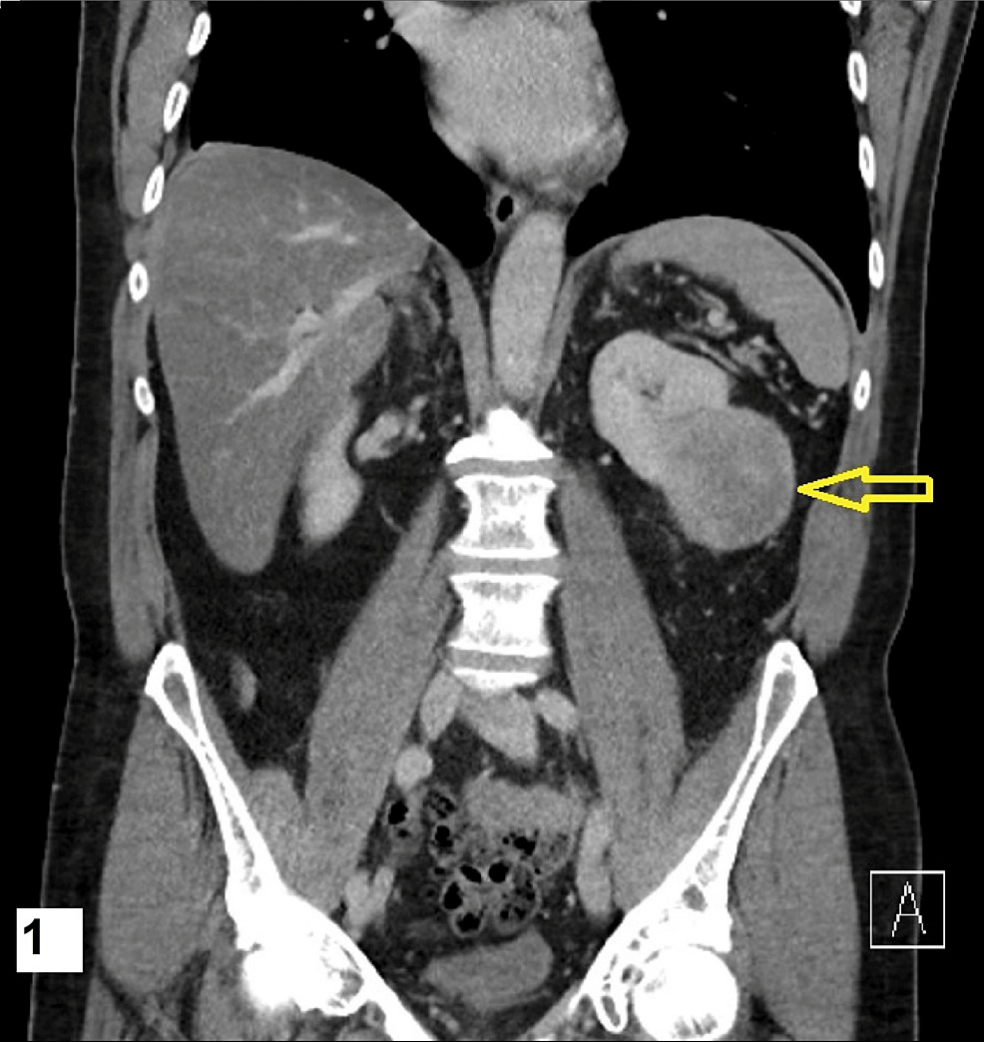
Coronal image of the CT scan showing the original tumour The yellow arrow points to the left kidney tumour.
